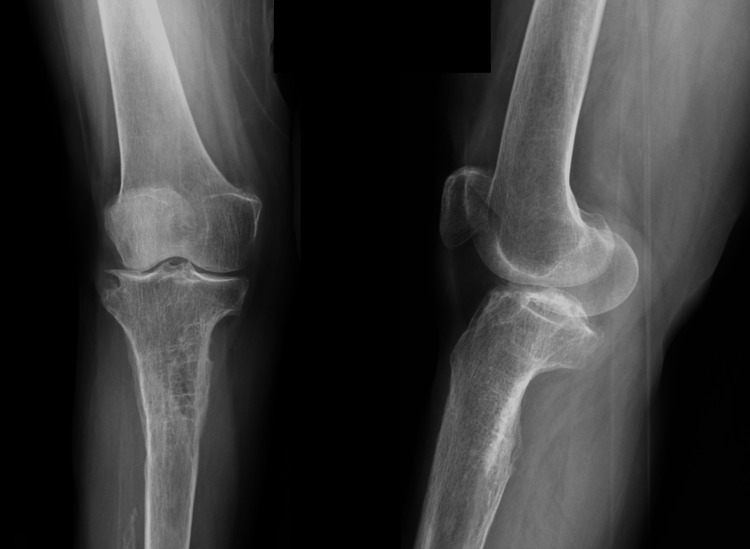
Plain radiographs showing lytic bone lesions in the proximal tibia (partially) and fibula. Note that the fibula is almost invisible on the plain radiographs.
